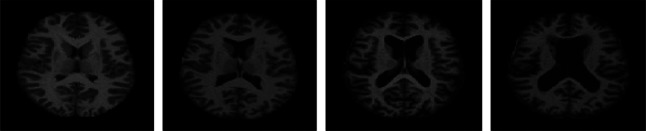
Sample images from each class of OASIS-MRI database
